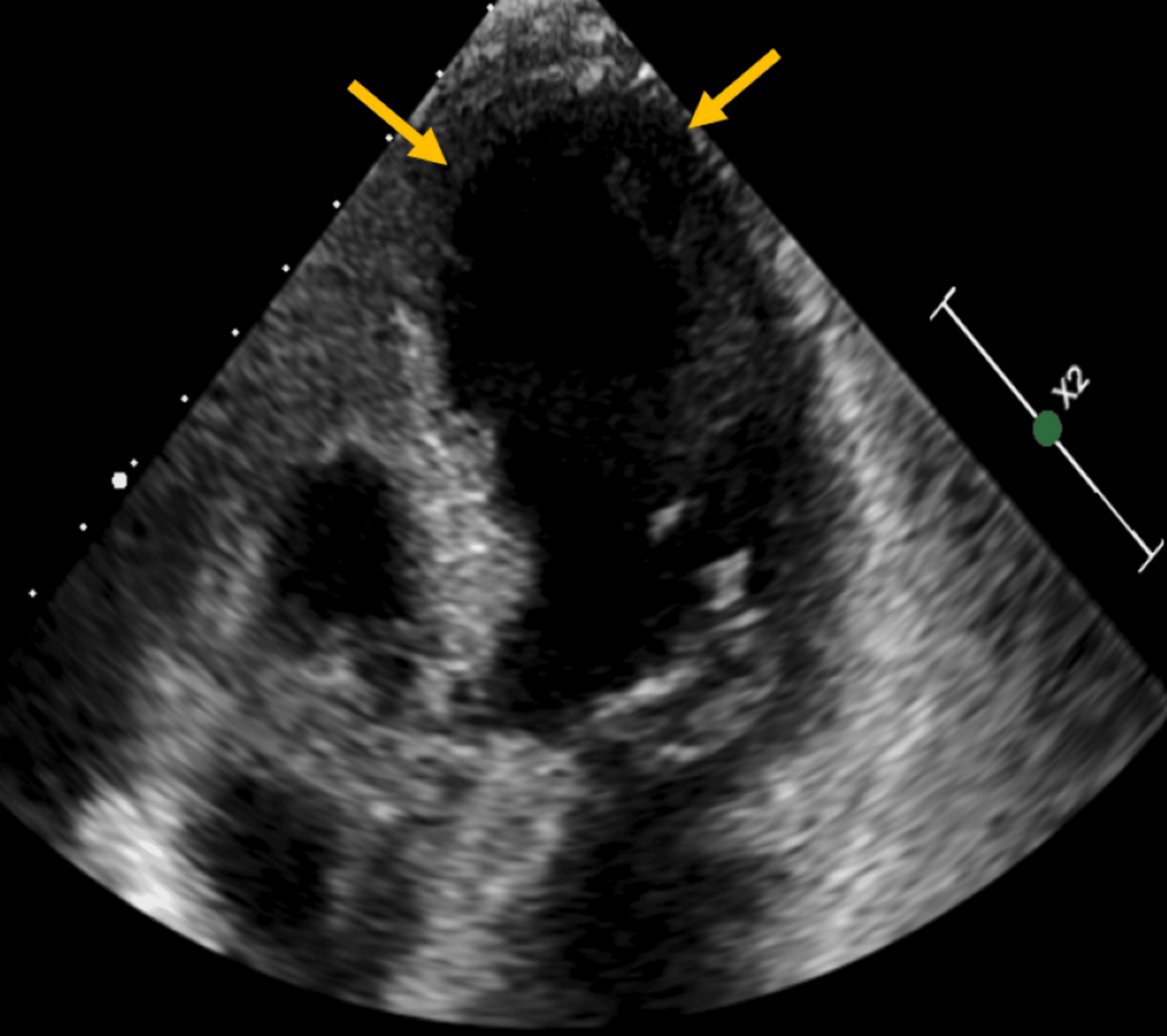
Echocardiogram on day 2 with hypokinetic apex and ejection fraction of 15%.
